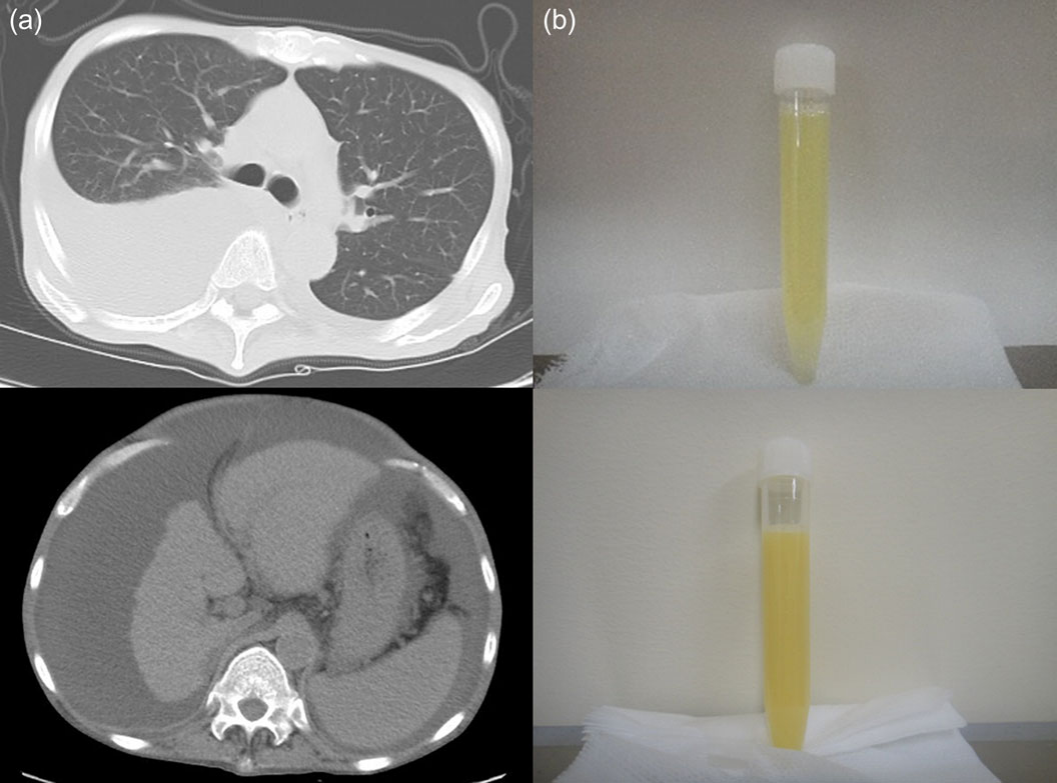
(a) CT imaging of the thorax and abdomen; (b) appearance of right pleural effusion (upper) and ascites (below).
